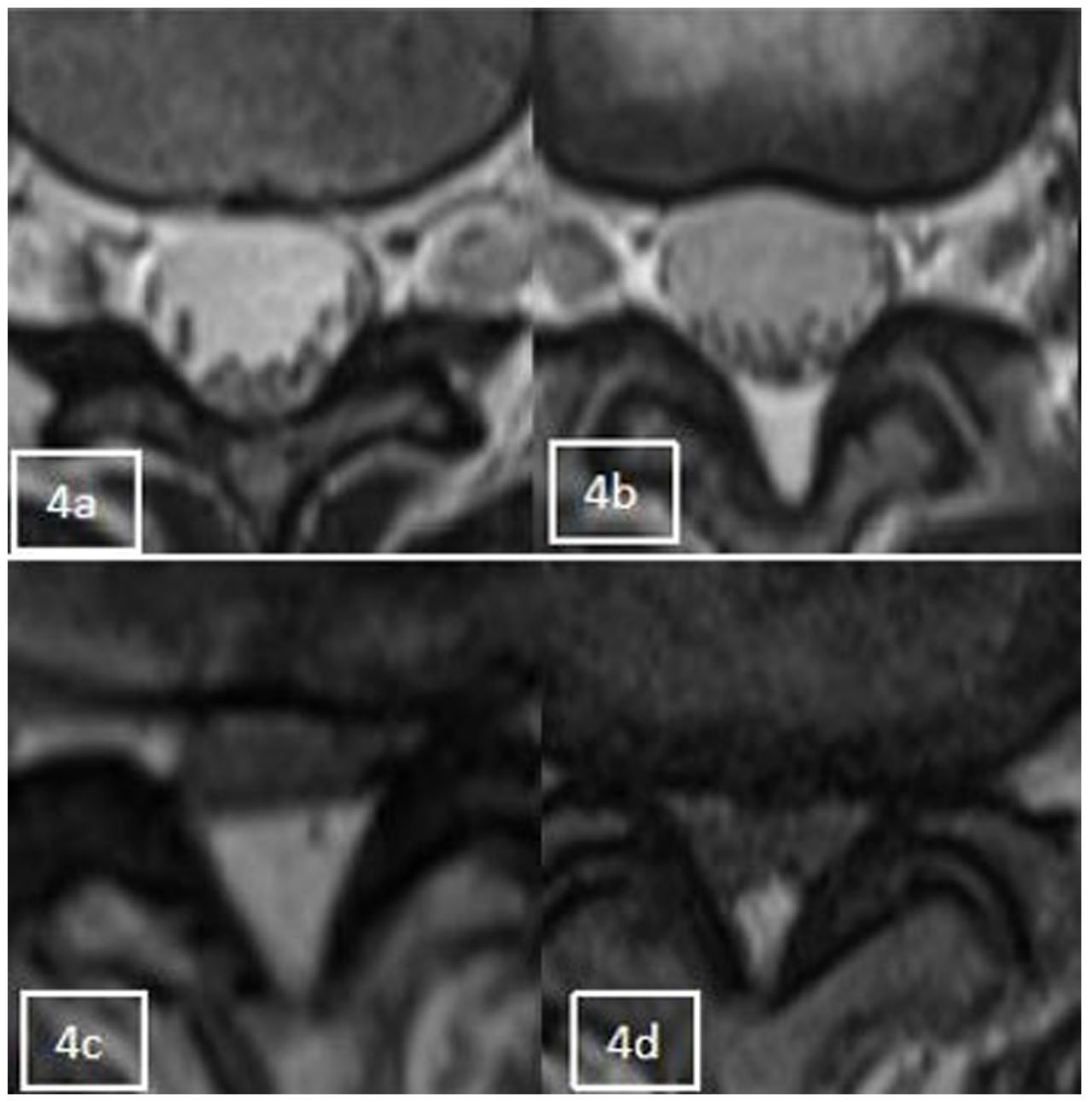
Axial appearance of epidural fat on T2-weighted MR images. Fat accumulation at each intervertebral disc level from L1–2 to L4–5 was classified as follows: absent (A), concave (B), flat (C), or convex (D).
